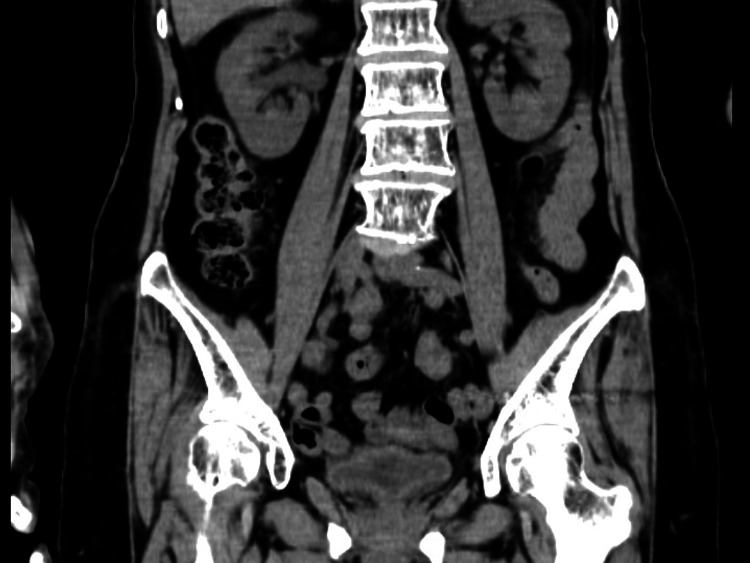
Abdominal computed tomography (coronal): showing no obvious abscess formation or fracture findings
